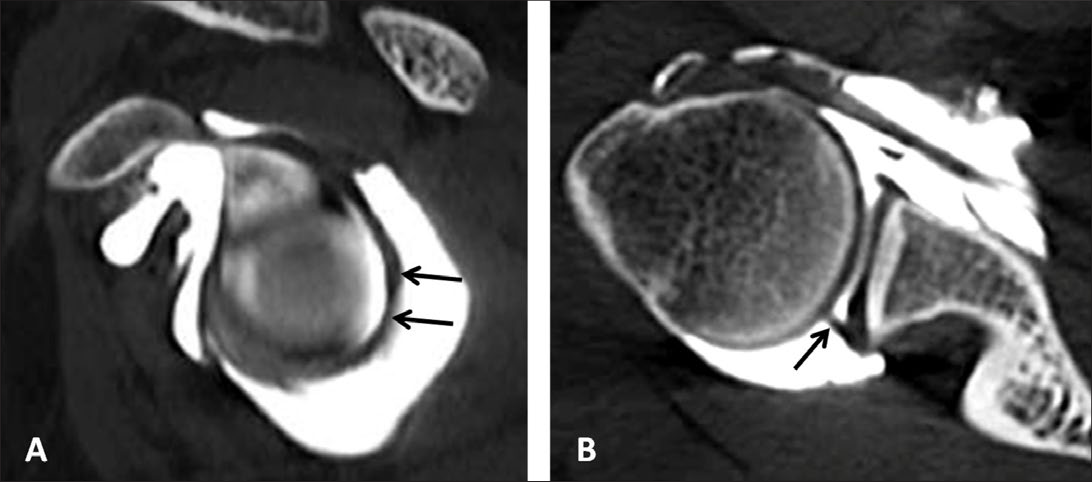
Posterosuperior glenohumeral ligament is demonstrated on (A) sagittal and (B) Axial CTA images (arrows, A and B). It arises from the posterosuperior part of the glenoid neck, medial to the posterosuperior labrum and the origin of the long tendon of the biceps. Laterally, it fuses with the posterior part of the rotator cable and fibers of the infraspinatus before these three structures jointly insert on the posterior facet of the greater tuberosity.
